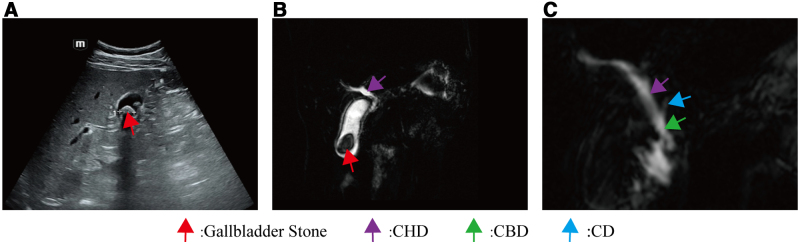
Preoperative abdominal ultrasound and MRI imaging studies. (A) Abdominal ultrasound indicates gallstones and gallbladder wall thickening. (B) MRCP confirmed signs of cholelithiasis and cholecystitis. (C) MRCP shows the cystic duct joining the common bile duct from the left side. CBD = common bile duct, CD = cystic duct, CHD = common hepatic duct, MRCP = magnetic resonance cholangiopancreatography, MRI = magnetic resonance imaging.
